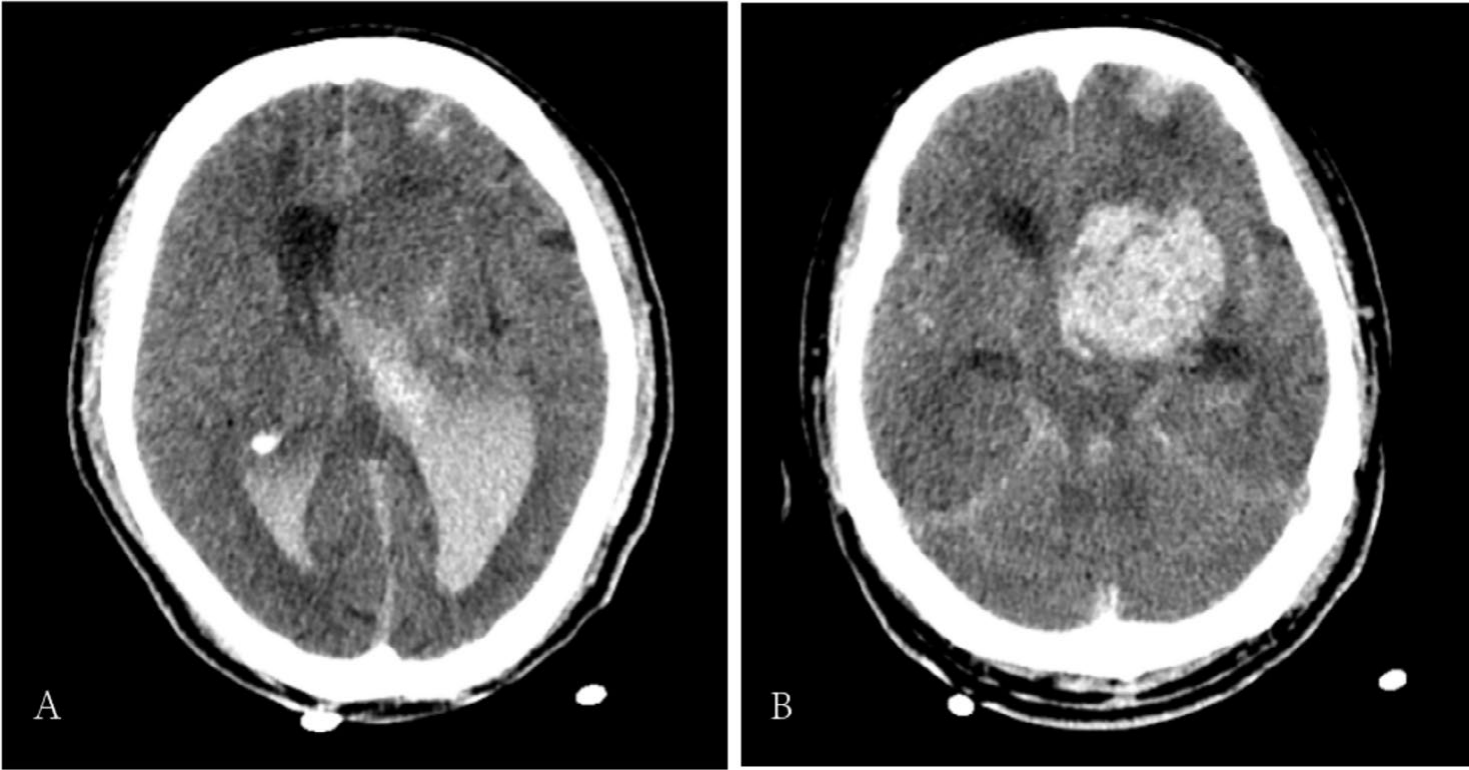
Postoperative cranial CT scan images: (A–B): Two hours after surgery, the cranial CT scan shows a mass‐like high‐density shadow in the left frontal lobe and basal ganglia region. The border is relatively clear, with a size of approximately 5.3 × 4.9 × 2.1 cm. Part of the mass has broken into the ventricles, and the ventricular system is enlarged, with a high‐density cast image observed inside. The midline structure is shifted approximately 0.7 cm to the right.
